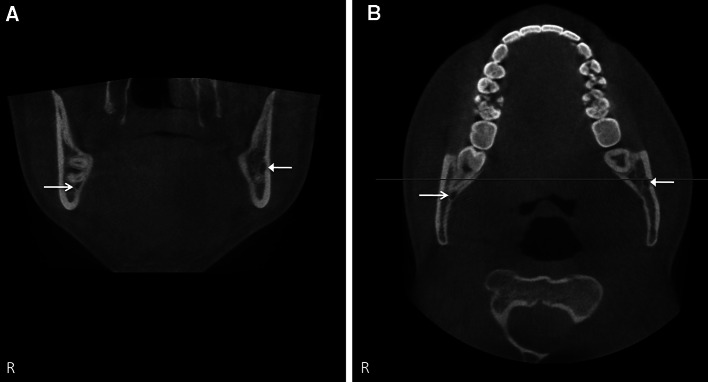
Coronal (a) and sagittal CBCT images (b) of impacted M3 right in close relation to the IANC (*arrow* on *right side*). The IANC on the *left side* is situated close to the buccal cortex (*arrow* on *left*
